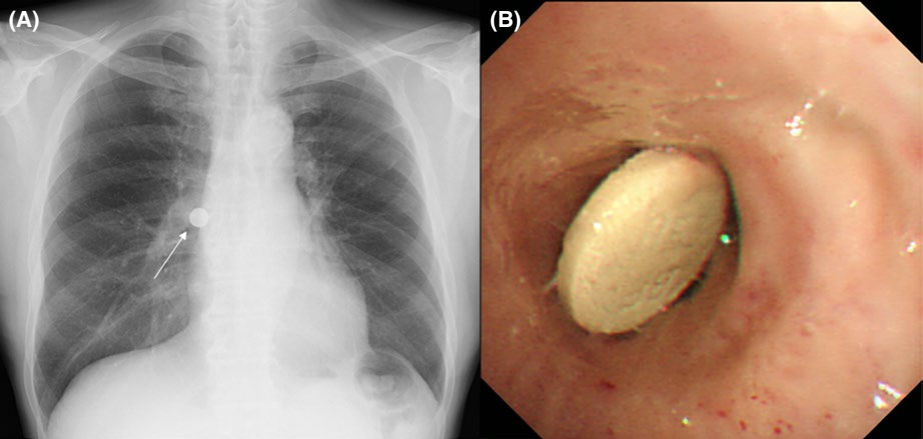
(A) Chest radiograph on admission, (B) bronchoscopic image showing foreign body in right bronchus intermedius.
